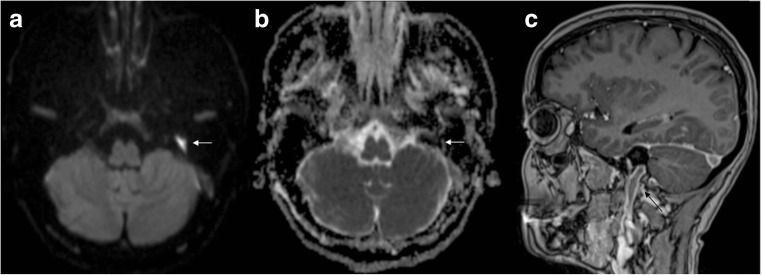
Venous thrombosis. DWI (a), ADC map (b) and sagittal reconstruction of T1 3D with gadolinium (c) show thrombosis of the left jugular vein (arrows), presenting with restricted diffusion and a filling defect in the vessel after contrast administration
